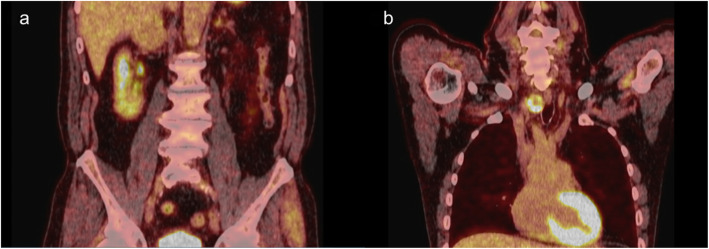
^18^F-FDG PET/CT: a. The right adrenal mass has mild homogeneous activity (maximum SUV of 2.7), which is similar to the activity to the liver. b. Posterior aspect of the right lobe of the thyroid gland is primarily peripherally FDG avid (maximum SUV of 12.7)
